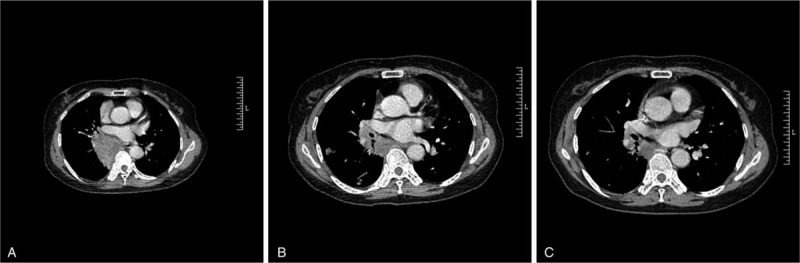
Chest CT scans on January 25, 2019 (A), March 26, 2019 (B), and April 19, 2019 (C). The tumor mass measured 6.5 cm × 5.3 cm, 2.9 cm × 2.3 cm, and 1.7 cm × 2.0 cm, respectively.
